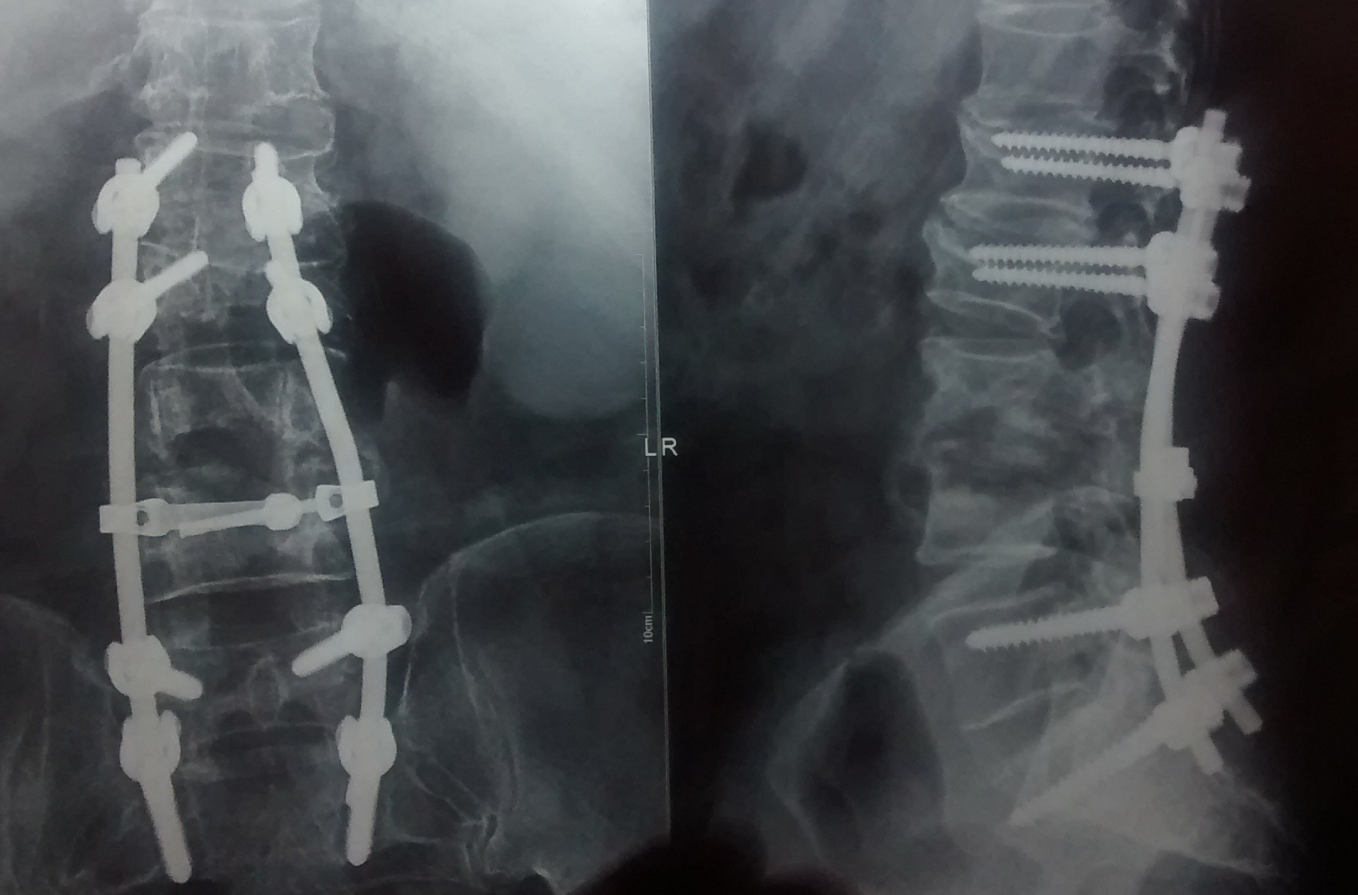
Postoperative X-rays at the two-year follow-up Postoperative X-rays at the two-years follow-up showing a stable construct
